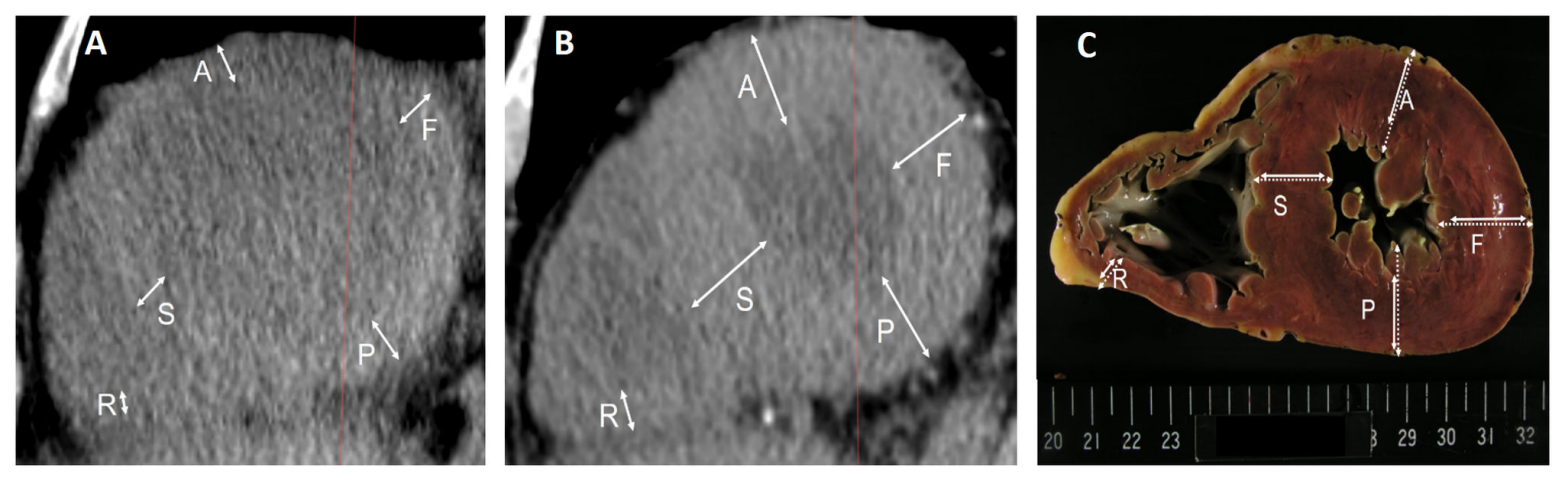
Ante mortem and postmortem measurements of the heart of a 60-year-old man. (a) Multiplanar reconstruction image of ante mortem CT. (b) Multiplanar reconstruction image of postmortem CT. (c) Photograph of the pathological cross-sectional slice. All three of these images represent the same plane. Solid lines represent measurements without including papillary muscles or epicardial fat, according to conventional methods. Dotted lines represent measurements with papillary muscles and epicardial fat. A, anterior wall of the left ventricle; F, left ventricular free wall; P, posterior wall of the left ventricle; S, ventricular septum; R, right ventricular wall.
